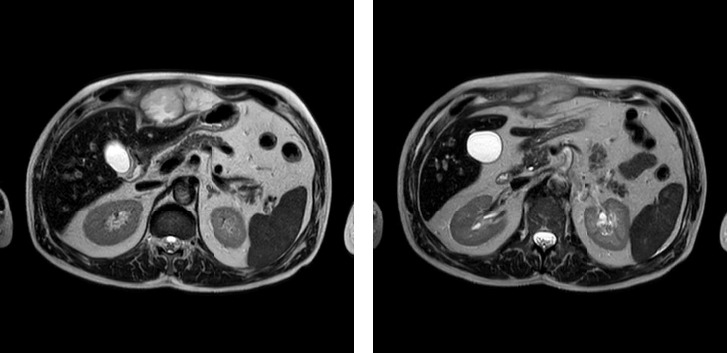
Single-shot fast spin echo T2 weighted magnetic resonance imaging showing a collection in the anterior abdominal wall located at the left paramedian region, which slightly imprints and displaces the left hepatic lobe (a). The content appears mildly and heterogeneously hyperintense on fluid-sensitive sequences, indicating a predominantly fluid component with proteinaceous content. The walls appear moderately thickened. The described finding appears compatible with an abscess collection. Moreover, there is a slight edema and inhomogeneity present in the visceral peritoneal fat (b).
